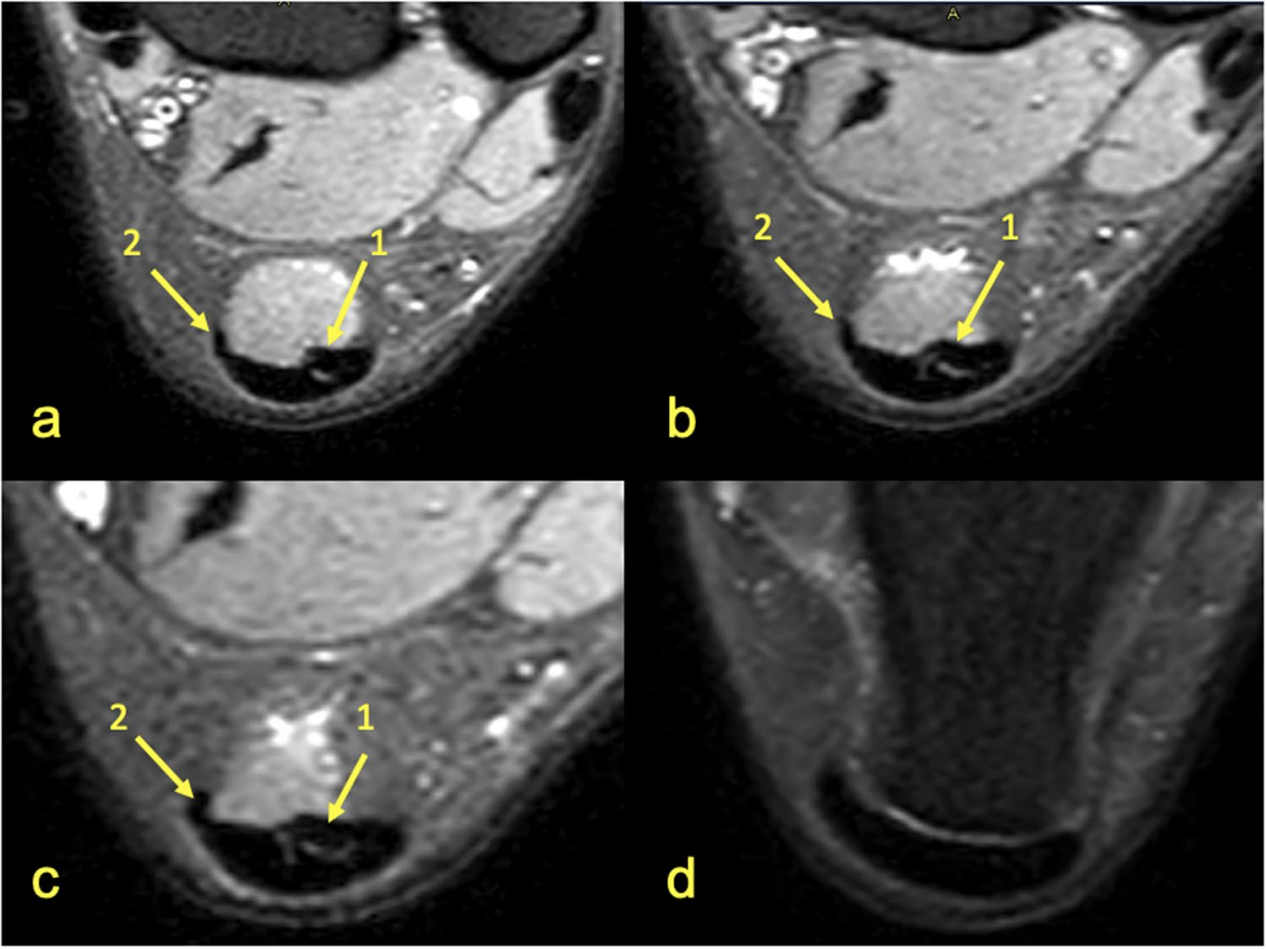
MRI left ankle, PD FS at the level of the distal tibia (a) is a slightly higher section than (b), the talus (c) and the calcaneus (d). A 37-year-old male with clinical suspicion of anterior conflict. The central tendon of the soleus muscle was absent. 1—S-LGC, 2—the plantaris tendon (type 4).
